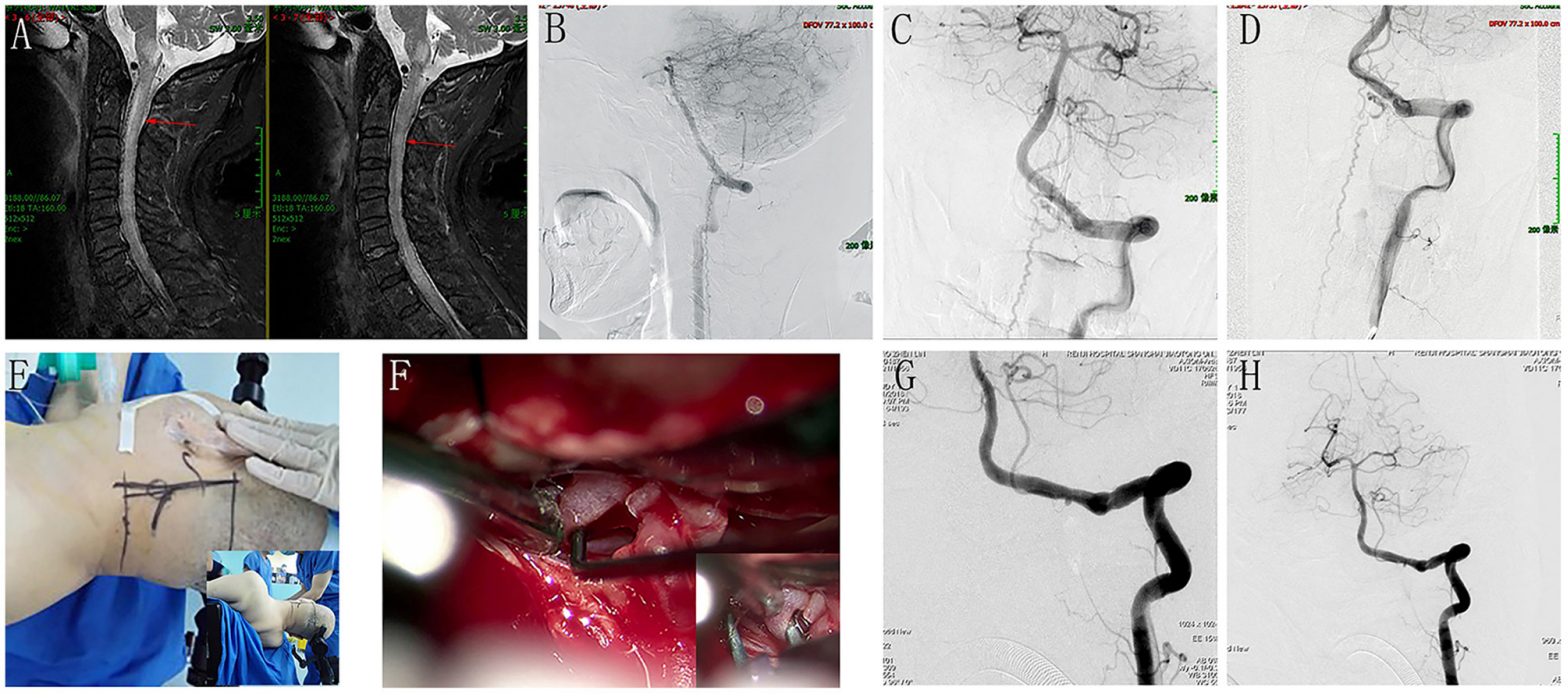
Perioperative neuroimages of Case 12. A cervical spine MRI showed an expanded spinal brain stem and a longitudinal extensive cervical cord enlargement with obvious edema, and vascular flow voids was also demonstrated in front of the cervical cord (A). The cerebral DSA demonstrated a DAVF located at the foramen magnum supplied by the meningeal branch of left VA, and the fistula was draining through the bridge-medullary veins into the dilated tortuous anterior spinal vein (B), lateral view; (C), anteroposterior view; (D), oblique view with magnification. (E) showed the incision line and the operation position (park bench position). F demonstrated a large tortuous vein was visible at the level of the foramen magnum, and it originated near the dural penetration of the left vertebral artery and formed a “C” pattern crawling between the vertebral artery and the medulla. (F) showed an aneurysm min-clip was temporarily applied to the origin of the fistula to occlude the shunt. Intraoperative DSA revealed the complete disappearance of the DAVF (G). After electrical coagulation and disconnection of the fistula at its origin from the dura, repeat cerebral DSA confirmed the complete obliteration of the fistula (H).
